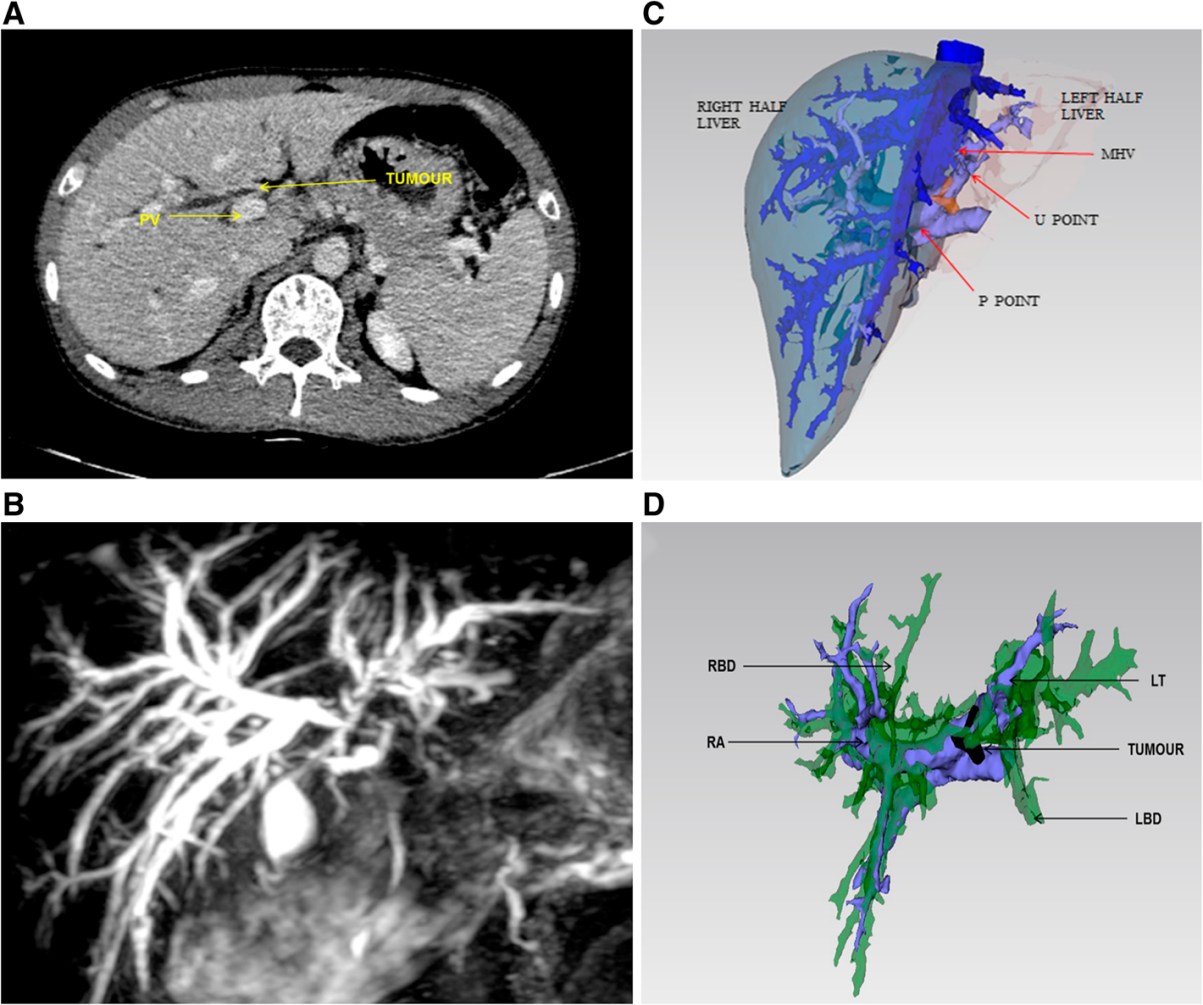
Case study of a single patient with preoperative 3D reconstruction. The patient was a 47-year-old male patient who had been suffering from yellowish urine and yellow sclera for 1 month. Preoperative TBil was 174 μmol/L and dbil was 147 μmol/L. a CT revealed that the left hepatic duct was mainly involved by the tumor, and the patient was accompanied by obstructive intrahepatic duct dilatation. b 3D reconstruction of the portal vein. c 3D visualization of the liver segments and the relationship between the tumor and intrahepatic ducts. d Relationship between the tumor and portal vein after the liver was concealed. *RA* right anterior portal vein, *LT* left portal vein, *RBD* right bile duct, *LBD* left bile duct
